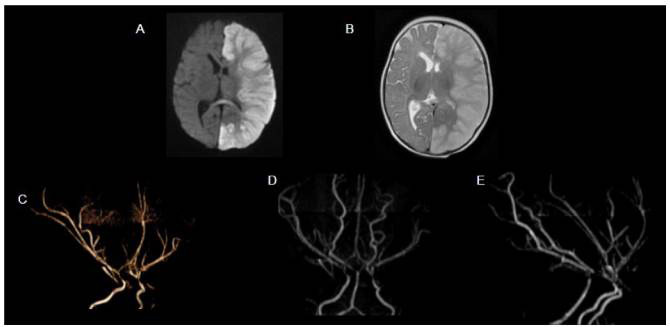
ACTA-2 mutation in 10-month-old female infant presenting with extensive left cerebral hemisphere infarct. DWI (A) shows a large hemispherical area of restricted diffusion. Axial T2WI (B) shows corresponding diffuse high signal intensity and mass effect. MRA with 3D rendering (C, D and E) demonstrates rectification of the intracranial arteries (loss of normal curvature) and tapering of distal internal carotid arteries.
